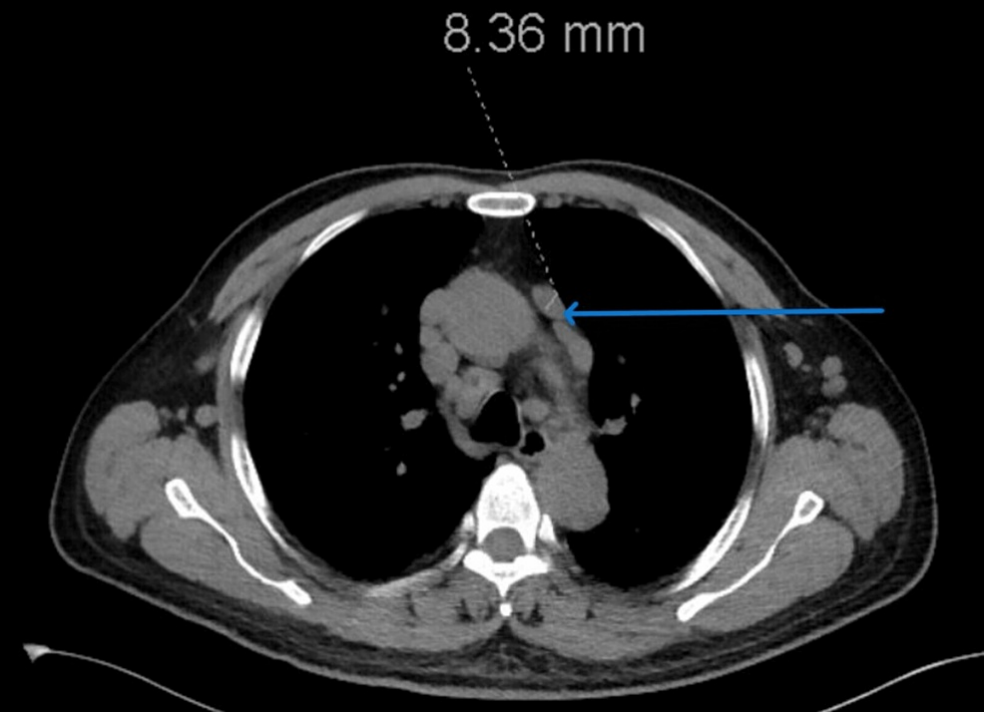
CT scan of the chest with mediastinal lymphadenopathy. CT: computed tomography.
